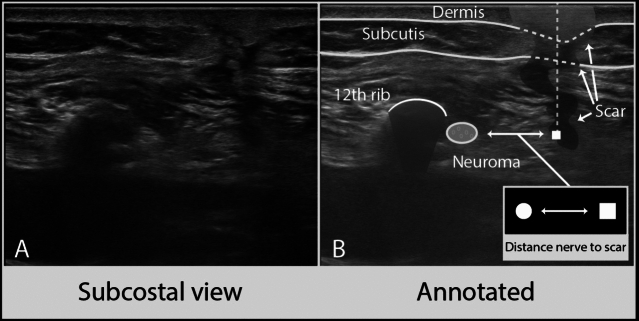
A, B Example of high-resolution ultrasound after 6 weeks with nerve damage, showing a neuroma of the subcostal nerve and distance to the surgical scar
